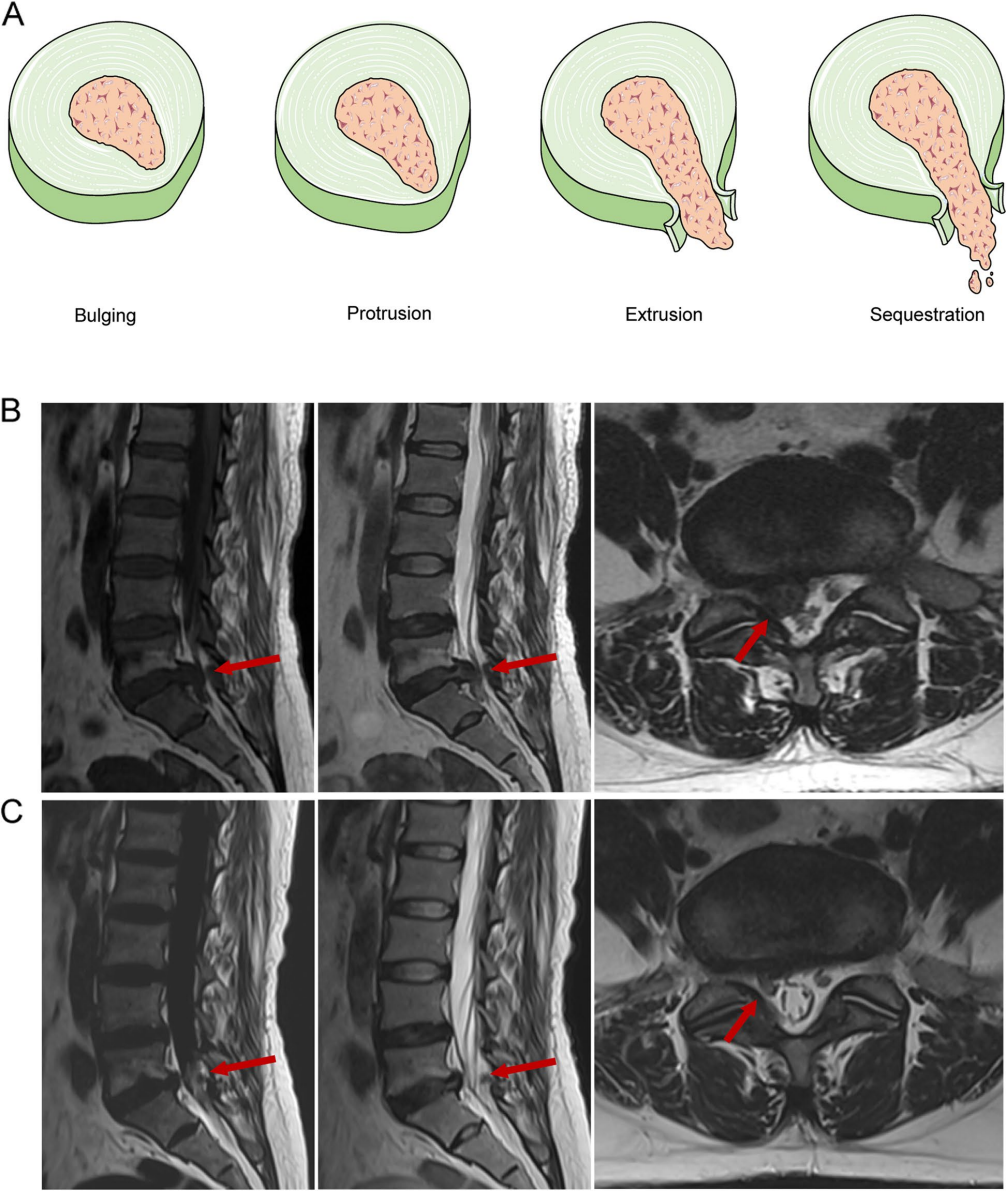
A The classification of LDH includes bulge, protrusion, extrusion, and sequestration. Elements in the image are taken from Servier Medical Art (https://smart.servier.com/). B Image from a 43-year-old female patient with LDH. The initial MRI showed that the L5/S1 intervertebral disc was hugely herniated, the center of the L5/S1 herniated intervertebral disc pushed the dural sac to the right, the right nerve root was compressed, and the dural sac was asymmetrically deformed. C After 1 year of conservative treatment, the L5/S1 disc herniation was significantly reduced, the protrusion was absorbed, and the dural sac was not significantly compressed or deformed
